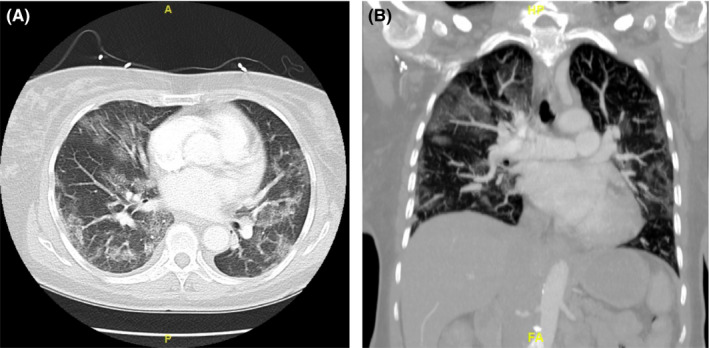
Computed tomography angiogram of the chest: diffuse bilateral subpleural ground‐glass opacities are seen consistent with the reported diagnosis of COVID pneumonia
